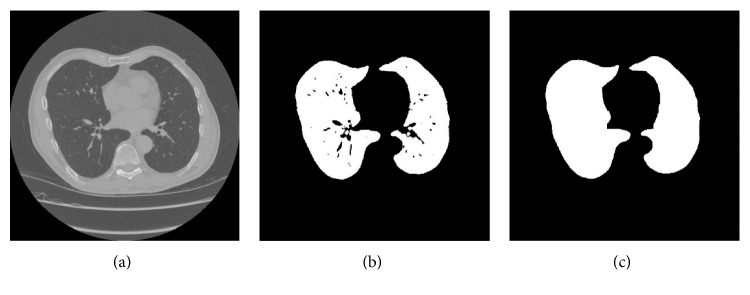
The final result of the extracted lung region: (a) original CT image, (b) lung tissue, and (c) the extracted lung region after filling holes.
